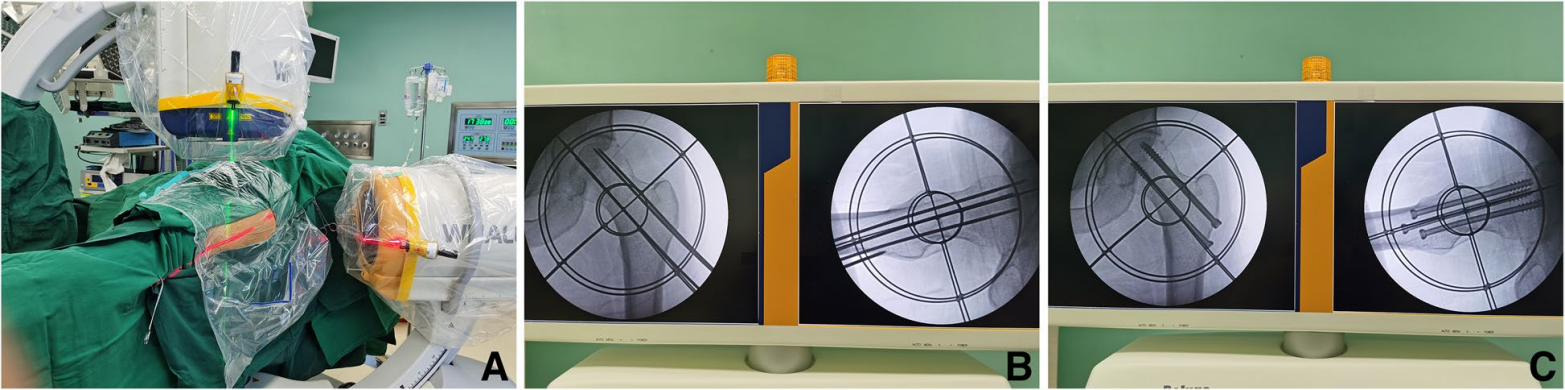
A The physical photos of the laser guiding navigation device. The horizontal red-light laser pointer and the coronal green-light laser pointer were fixed to the G-arm’s image intensifier by the special round strap. The angle of the laser pointer was determined by the position of the two positioning rings. B X-ray fluoroscopy confirmed that the Kirschner wires were inserted in the ideal position by laser. C X-ray fluoroscopy confirmed that the femoral neck cannulated screws were Inserted
